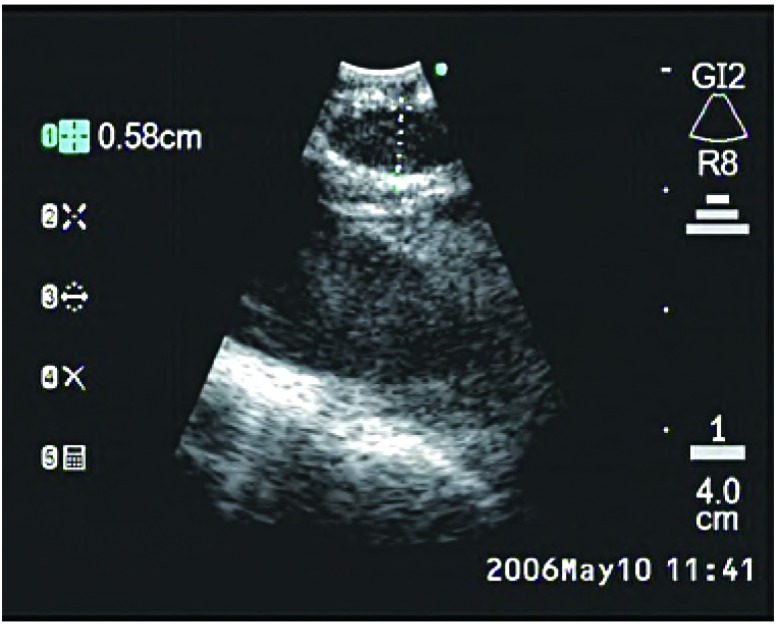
支气管内超声所见右侧气管旁淋巴结 Endobronchial ultrasound of right paratracheal node
